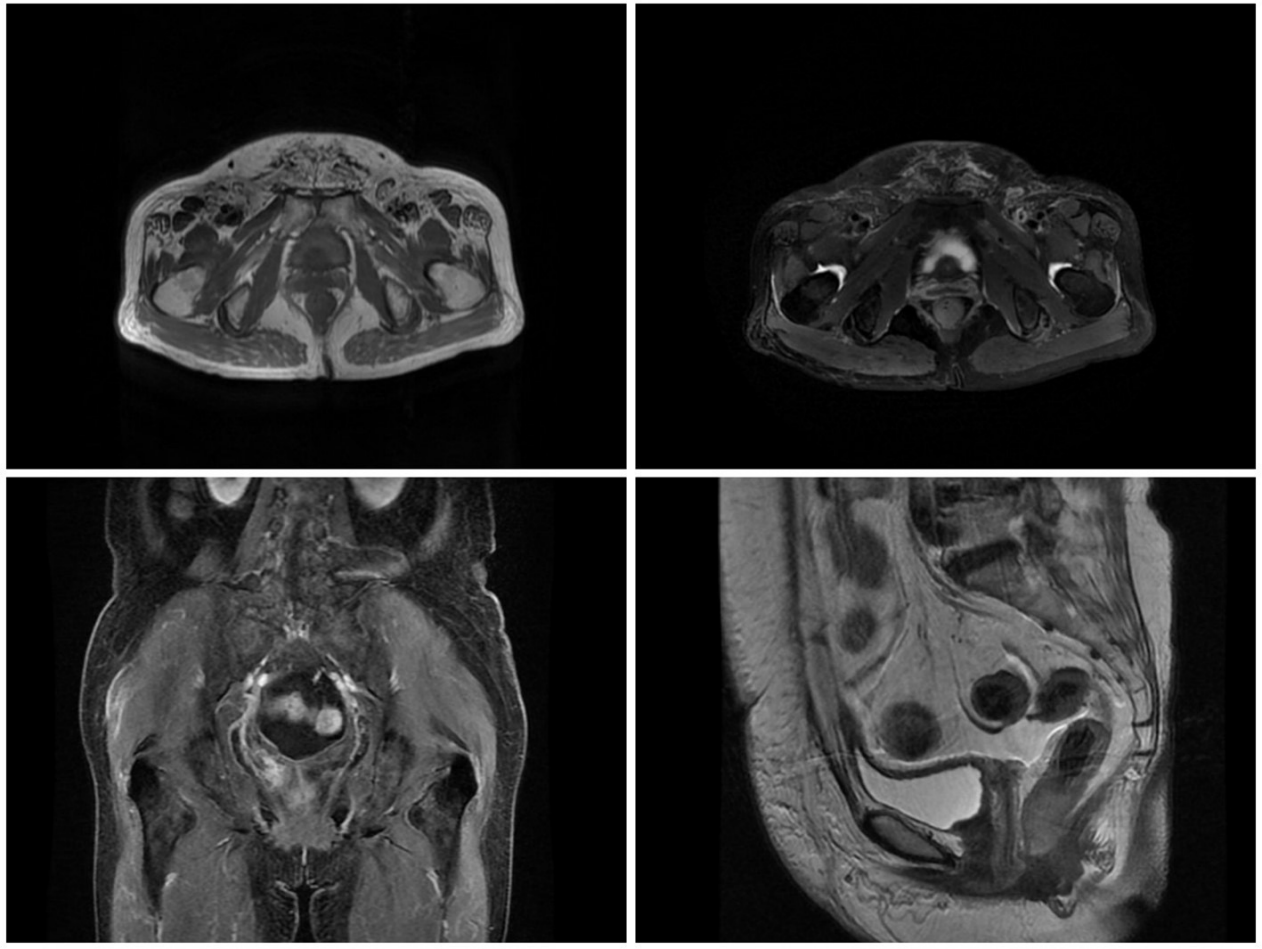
The pelvic MRI on 1 month after surgery showed no residual tumor tissue or metastasis.
